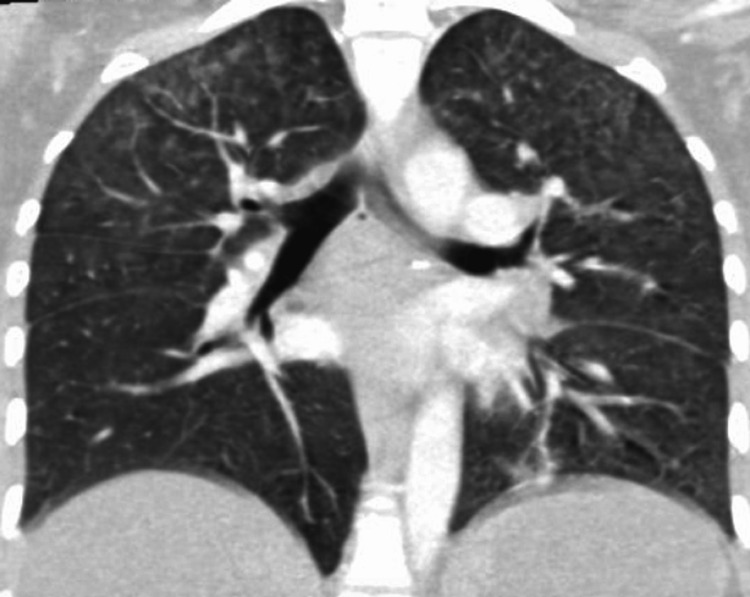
CT pulmonary angiogram (CTPA) showing ground glass nodules suggestive of hypersensitivity pneumonitis
